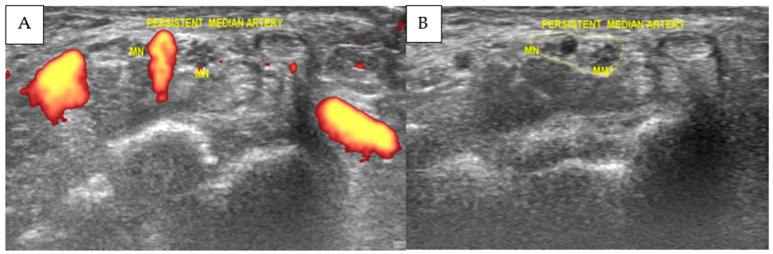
Transverse ultrasound image of the carpal tunnel showing a bifid median nerve (BMN) and a persistent median artery (PMA) with loss of the normal fascicular pattern (A). The combined cross-sectional area of the BMN was 0.19 cm^2^ (19 mm^2^), calculated as the sum of both nerve trunks (B). Power Doppler confirmed vascular flow within the PMA. MN, median nerve; PMA, persistent median artery.
